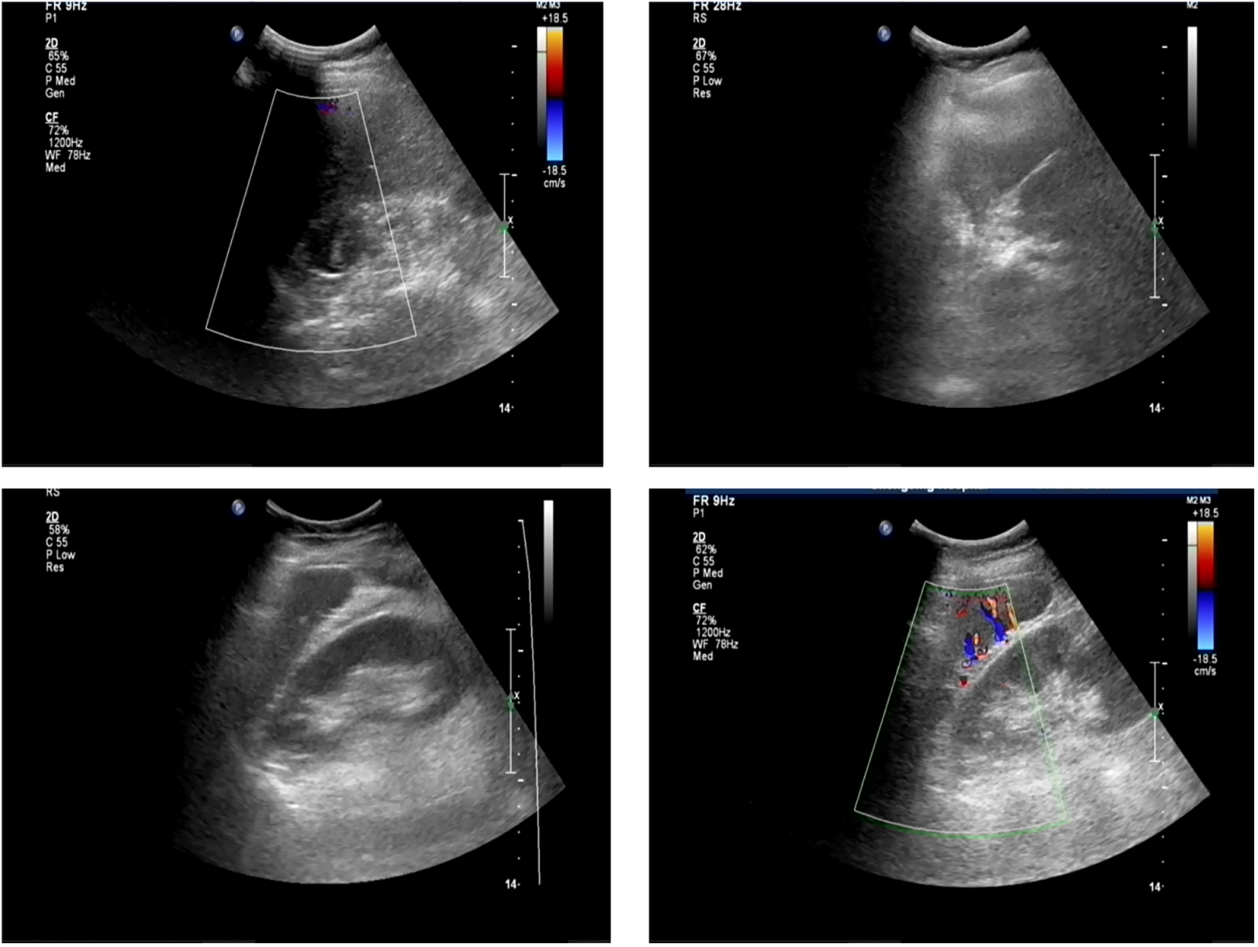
Ultrasound showing normal bilateral adrenal glands.
